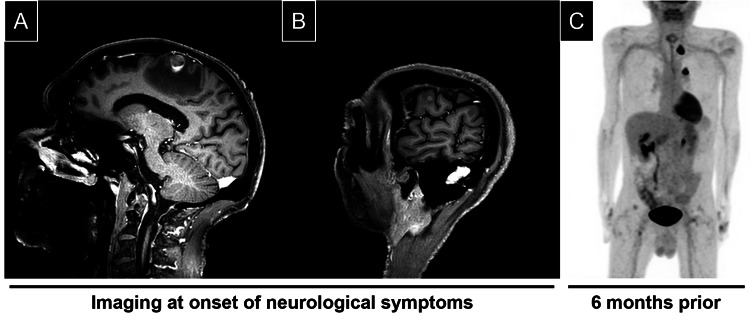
Brain lesions identified in patient with history of non-malignant lung nodules Magnetic resonance imaging (MRI) of brain lesions with significant surrounding edema (A-B). Lesions identified in the right superior frontal gyrus measuring 1.5 x 1.2 x 1.3 cm (A) and in the right precentral gyrus measuring 0.5 x 0.5 x 0.5 cm (B). Long-axial positron emission tomography (PET) scan six months prior demonstrated high physiological uptake in lung nodules, which was interpreted as non-malignant (C).
